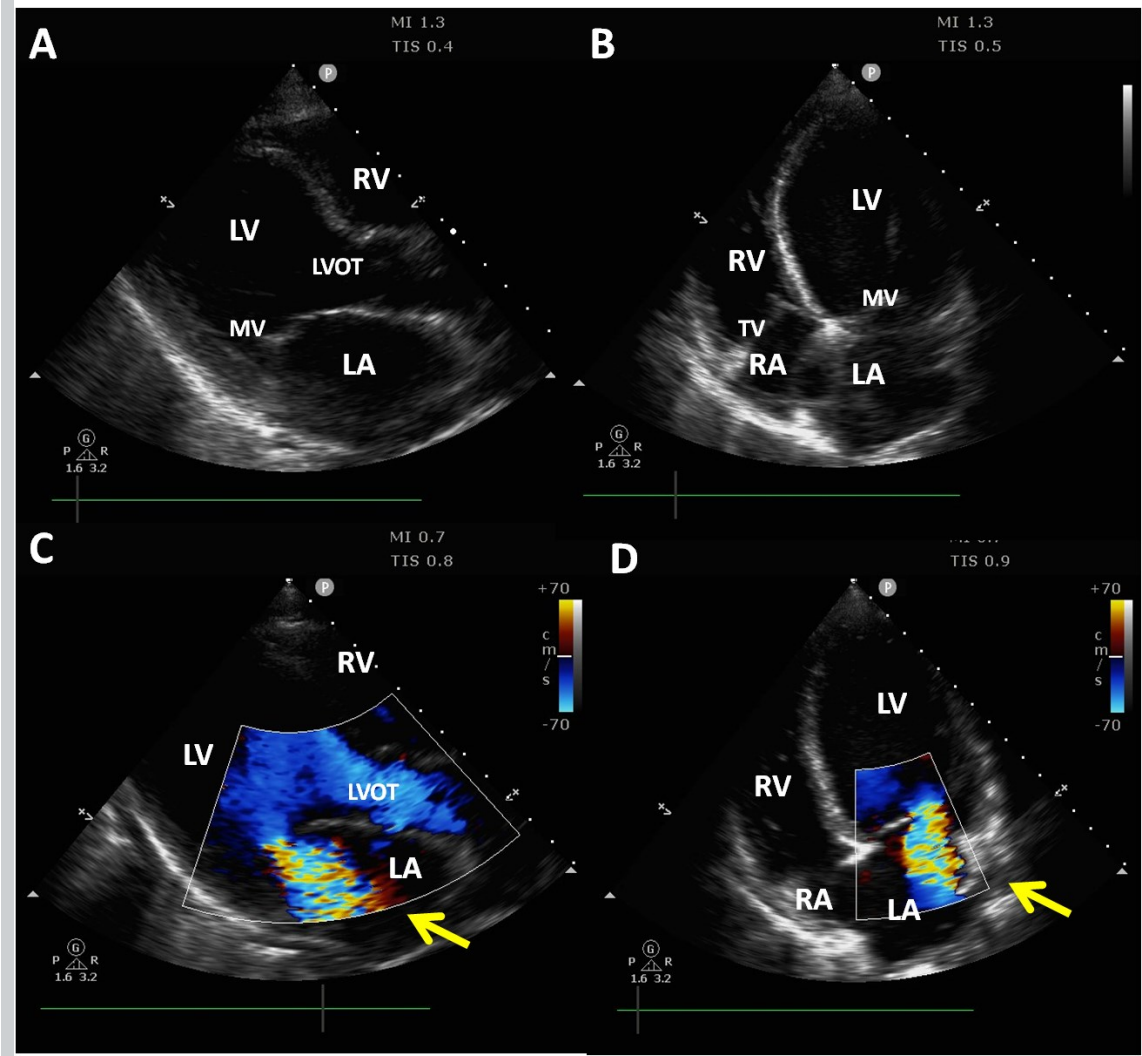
Parasternallong axis (A) and apical 4-chamber (B) views showing a dilated left ventricle(LV). Similar views are shown in panels (C) and (D), respectively, with colorDoppler demonstrating significant mitral regurgitation (yellow arrow). RV =Right ventricle; LVOT = Left ventricular outflow track; RA = Right atrium; MV =Mitral valve; TV = Tricuspid valve.
